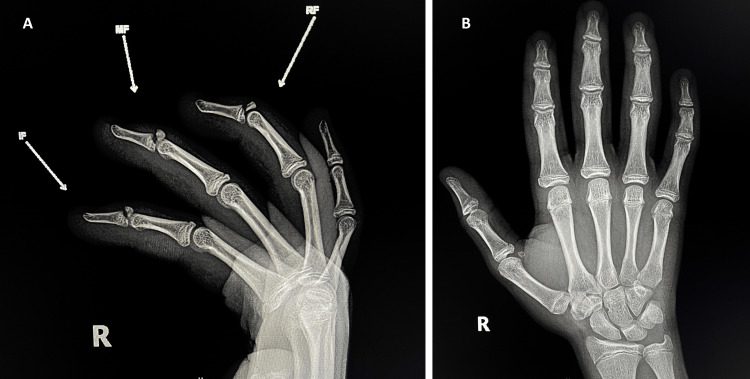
Right hand x-ray of a 14-year-old boy showing multiple bony mallet finger injuries at the base of the second, third, and fourth distal phalanges (white arrows). (A) Lateral view, (B) Anteroposterior view. Abbreviations: IF, index finger; MF, middle finger; RF, ring finger.
